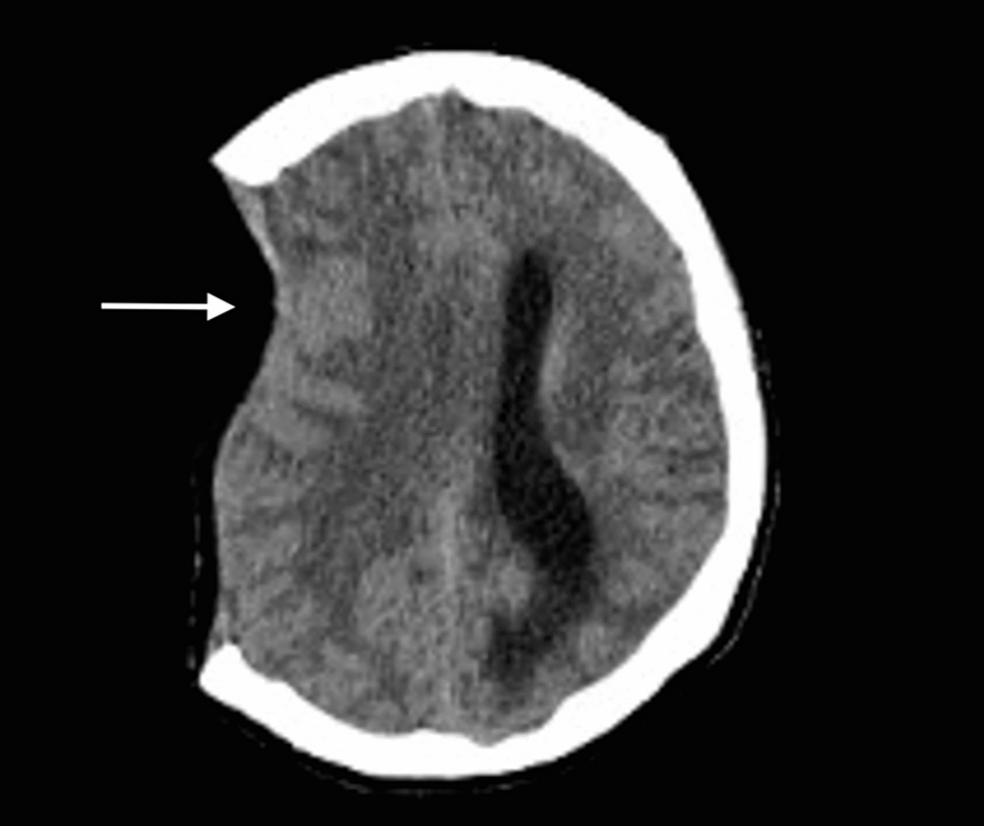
Computed tomography scan interval development of sunken flap syndrome with 11 mm of a leftward midline shift, crowding of the perimesencephalic cisterns, and left lateral ventricular trapping (white arrow)
